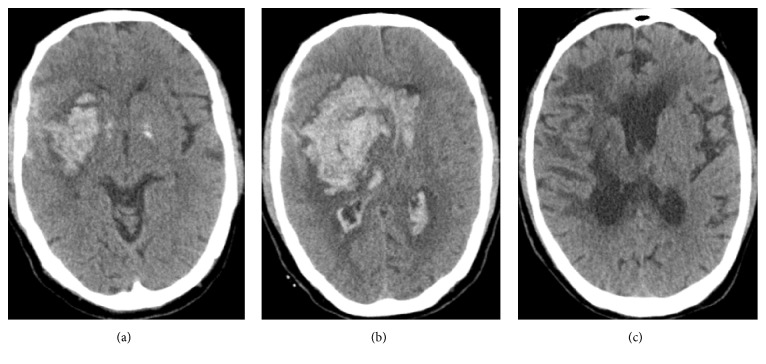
Cranial CT scan of patients with ICH showed Blend Sign II. (a) Hematoma volume is about 20 ml on admission. (b) Cranial CT scan shows that volume of hematoma was 83 ml on 1 hour after admission. (c) GOS of patient was 2 at discharge.
